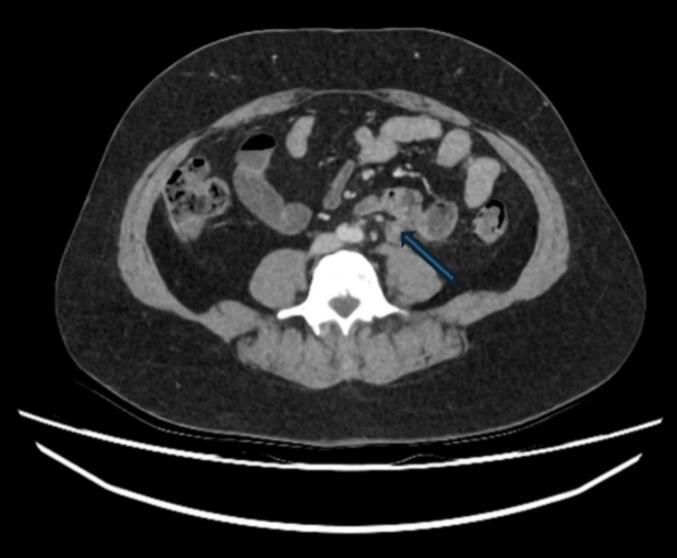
Axial contrast-enhanced CT image demonstrating distended poorly enhancing left ovarian vein (arrow).
